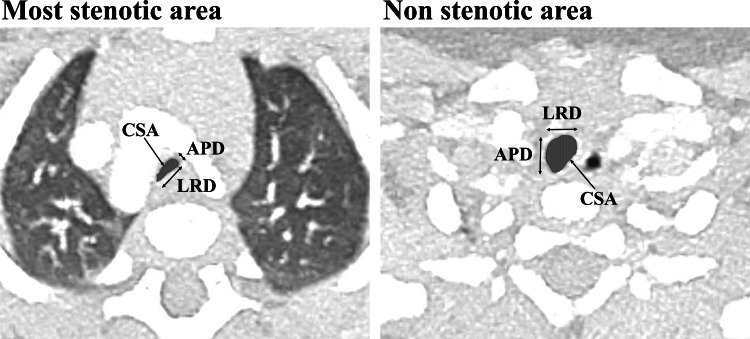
Airway measurement methods Lumen diameters (APD, LRD, CSA) were measured in axial cross-section in the pulmonary window setting of contrast-enhanced computed tomography. The tracheal lumen is indicated by vertical stripes. APD, anterior-posterior diameter; CSA, cross-sectional area; LRD, left-right diameter
